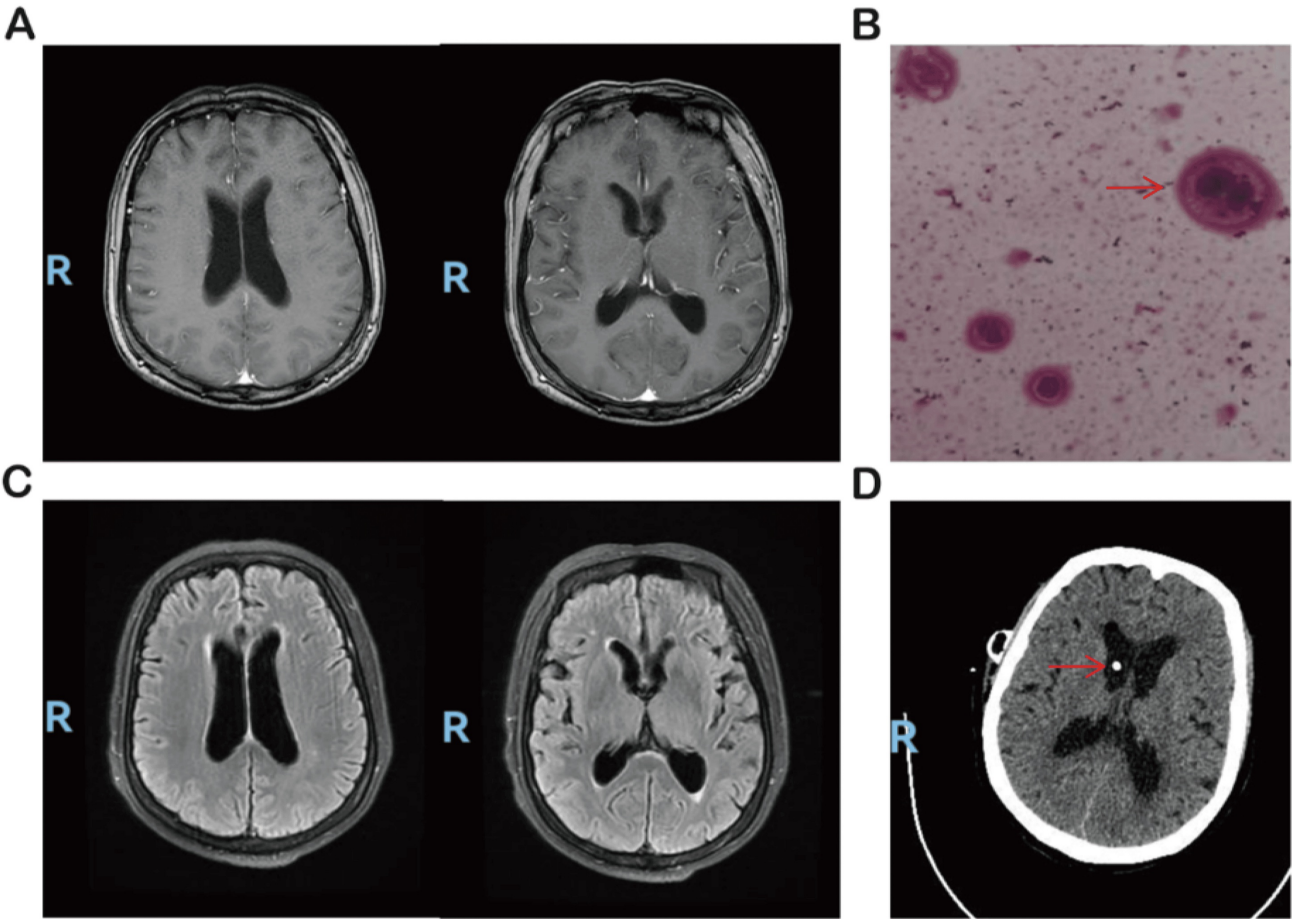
Neuroimaging and cerebrospinal fluid findings associated with leptomeningeal metastases. (A) Brain MRI on January 1, 2025, revealed no significant abnormalities. (B) Cerebrospinal fluid cytology showed large atypical cells with abundant cytoplasm and eccentrically located, hyperchromatic nuclei. (C) After initiating furmonertinib 240 mg daily, the patient’s dizziness improved; follow-up MRI on April 14, 2025, remained unremarkable. (D) On May 19, 2025, cerebrospinal fluid diversion was performed to relieve intracranial hypertension; high-density shadow in the ventricle represents the drainage catheter.
